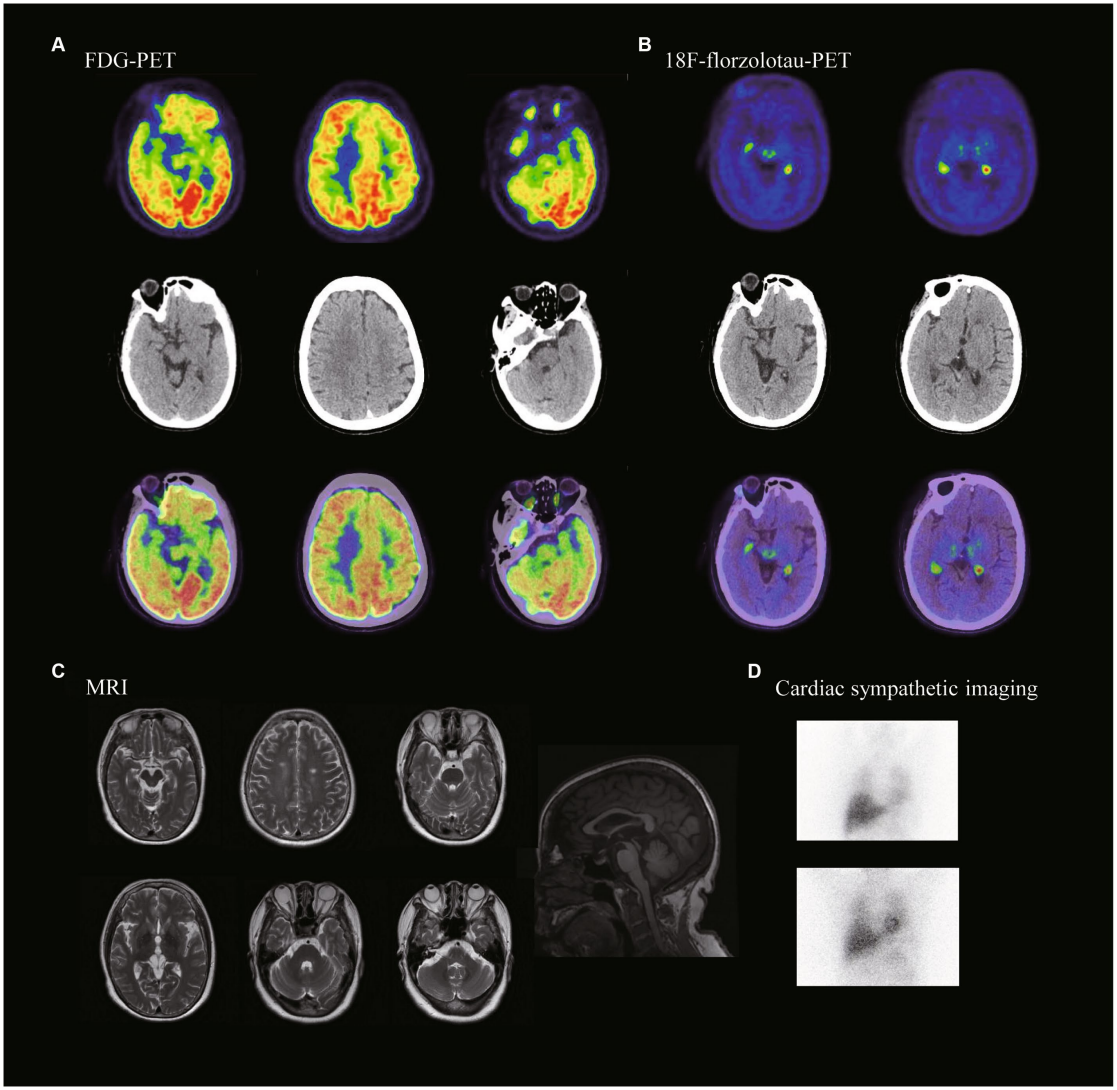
Neuroimaging of the patient with MAPT variant. (A) FDG-PET showed hypometabolism in the midbrain, left prefrontal lobe and bilateral temporal poles. (B) 18F-florzolotau-PET showed tau protein deposition seen in bilateral thalamus and brainstem. (C) MRI showing midbrain atrophy. (D) Cardiac sympathetic imaging showed no damage to cardiac sympathetic nerves. (15 min heart/mediastinum ratio (H/M ratio) = 2.56; 4 h heart/mediastinum ratio (H/M ratio) = 2.63).
